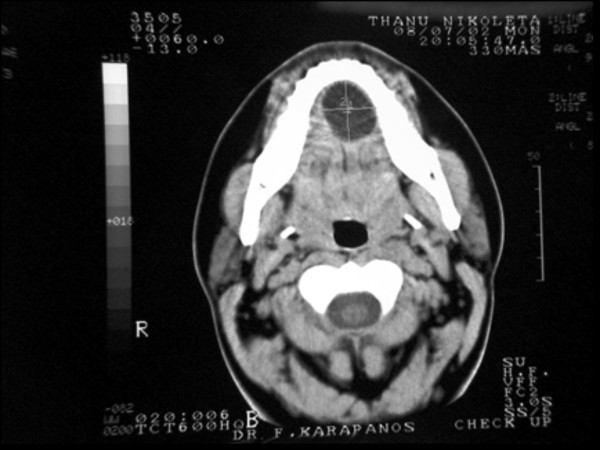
Axial CT section of the floor of the mouth showed a 1.38 × 1.18 in hypodense, non-enhancing mass located within the sublingual space, over the geniohyoid muscle.
